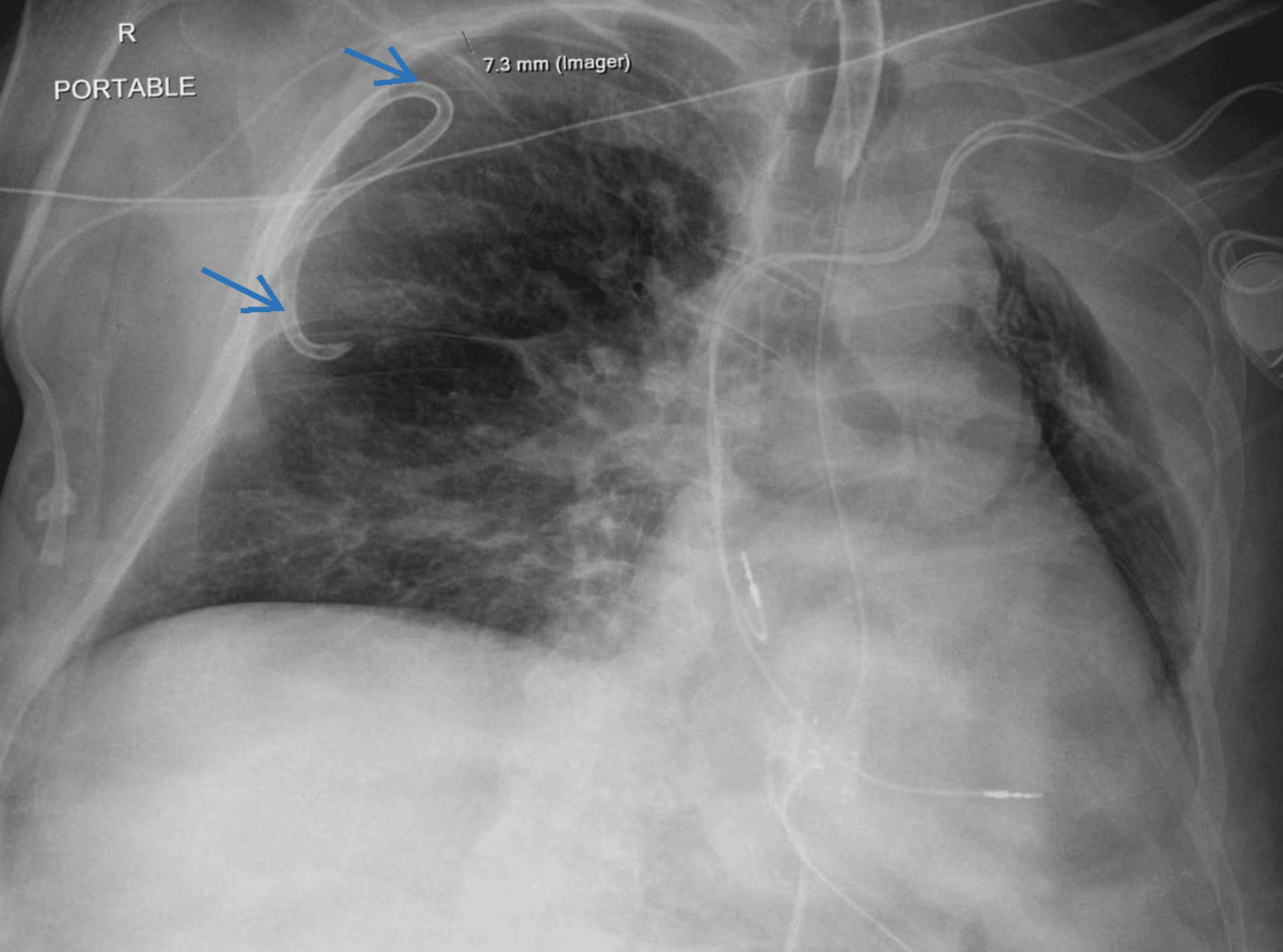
Postoperative chest X-ray Arrows show appropriate chest tube positioning and resolution of pneumothorax.
